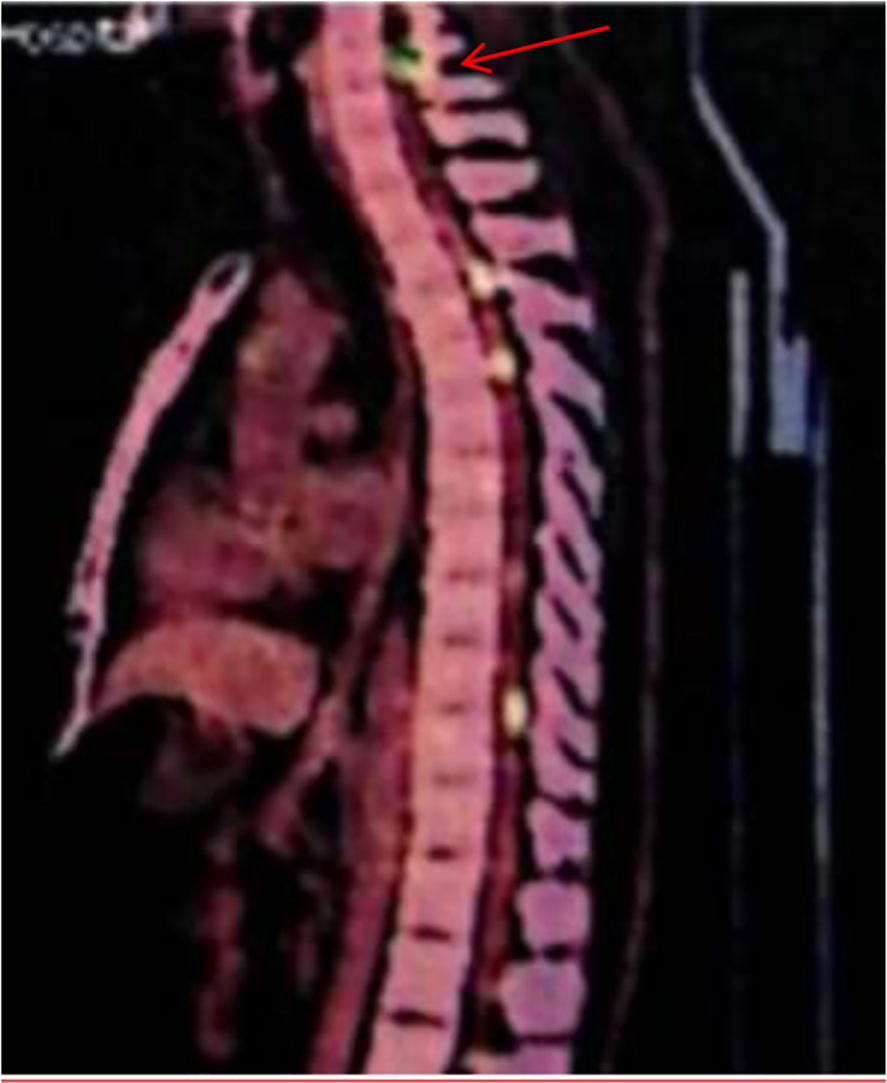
PET/CT: Multiple abnormally high metabolic signals were detected in the spinal cord.
